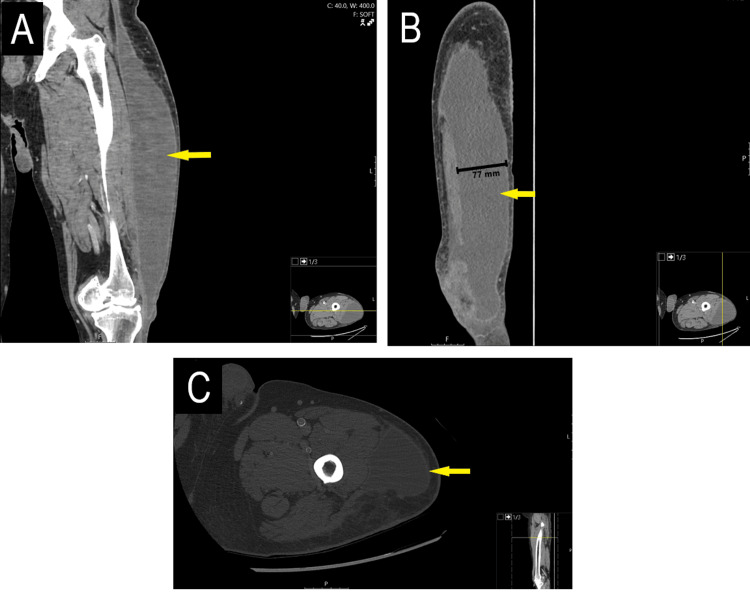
CT scan of the left lower extremity soft tissue with contrast during February hospitalization A) CT coronal lower bone with contrast showing the fluid collection. B) The collection measured 7.4 x 7.7 cm. C) Lower extremity soft tissue axial view showing collection of fluid. There is surrounding soft tissue edema in the fat of the left gluteal region.
